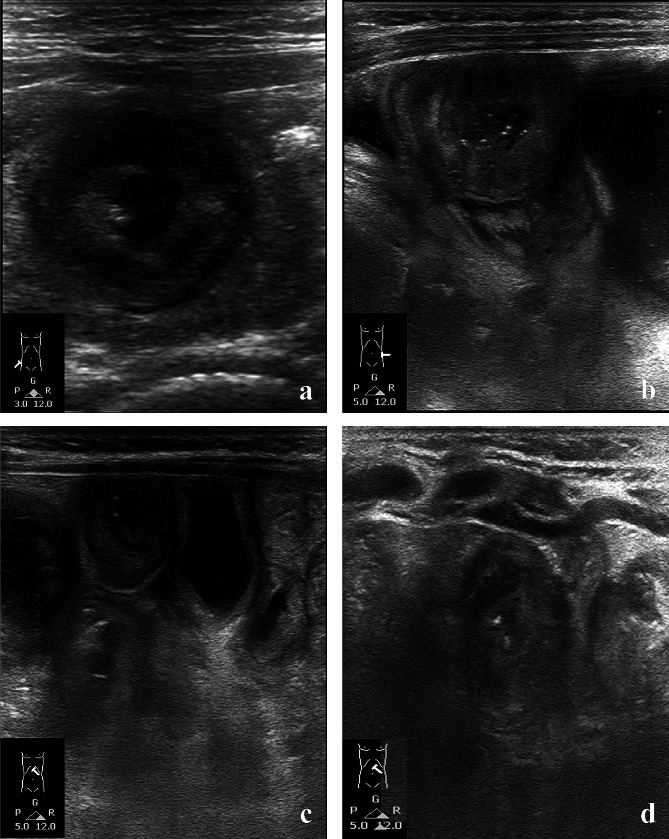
Diverse recurrent loci of recalcitrant intussusception on sonography. (a) Initial image of right lower quadrant (b) Subsequent image of left middle quadrant (c) Third image of left upper quadrant (d) Fourth image of left upper quadrant
